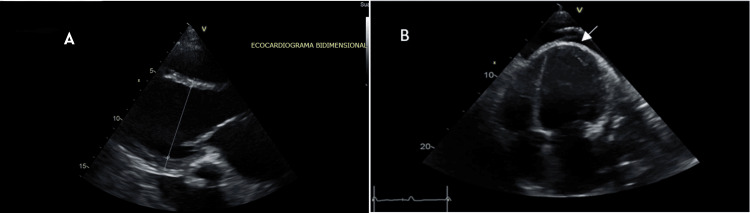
Two-dimensional transthoracic echocardiography (A) Parasternal long-axis echocardiographic view demonstrating a dilated left ventricle. (B) Apical four-chamber echocardiographic view demonstrating biatrial enlargement, thickened pericardium, and a small pericardial effusion (8 mm), without signs of tamponade physiology.
